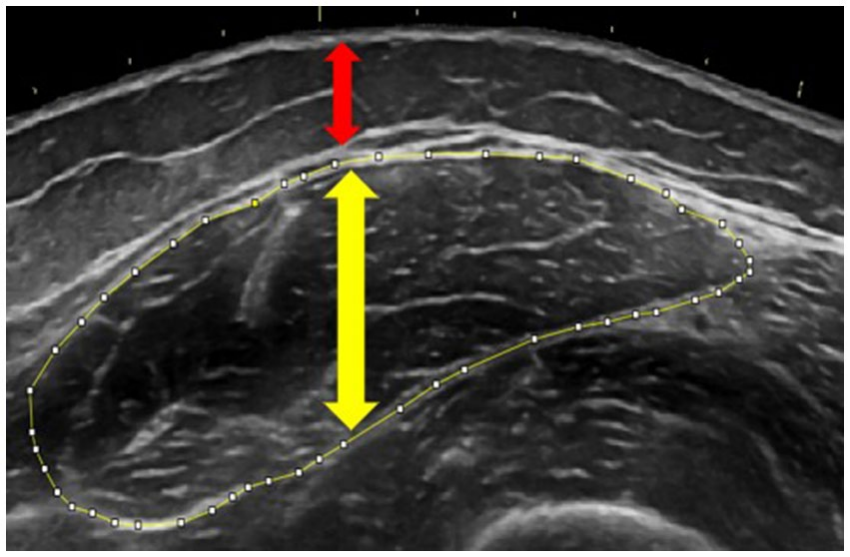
Ultrasonography image demonstrating measurements of muscle cross-sectional area (outlined in yellow), muscle thickness (yellow arrow), and subcutaneous adipose thickness (red arrow) of the rectus femoris. Tracing was performed using ImageJ software.
